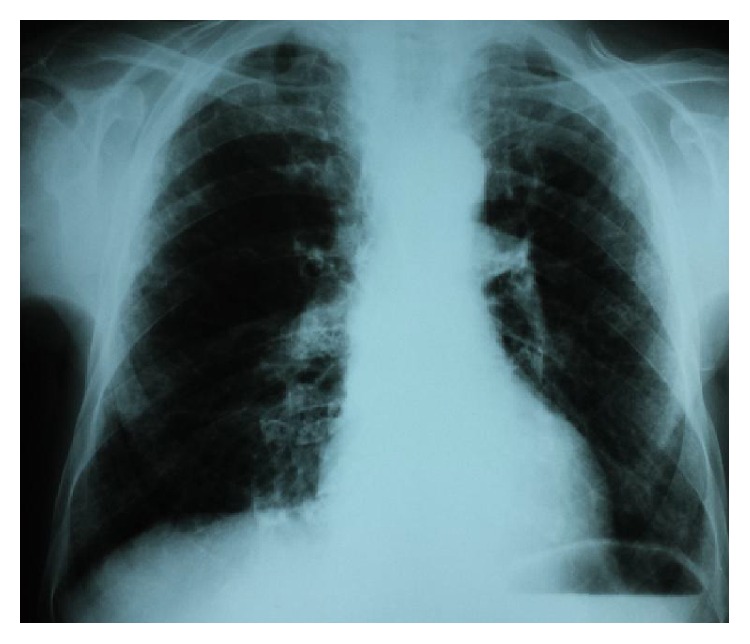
Chest X-ray conducted 24 years ago, displaying a trachea enlargement without any signs of fibrosis.
